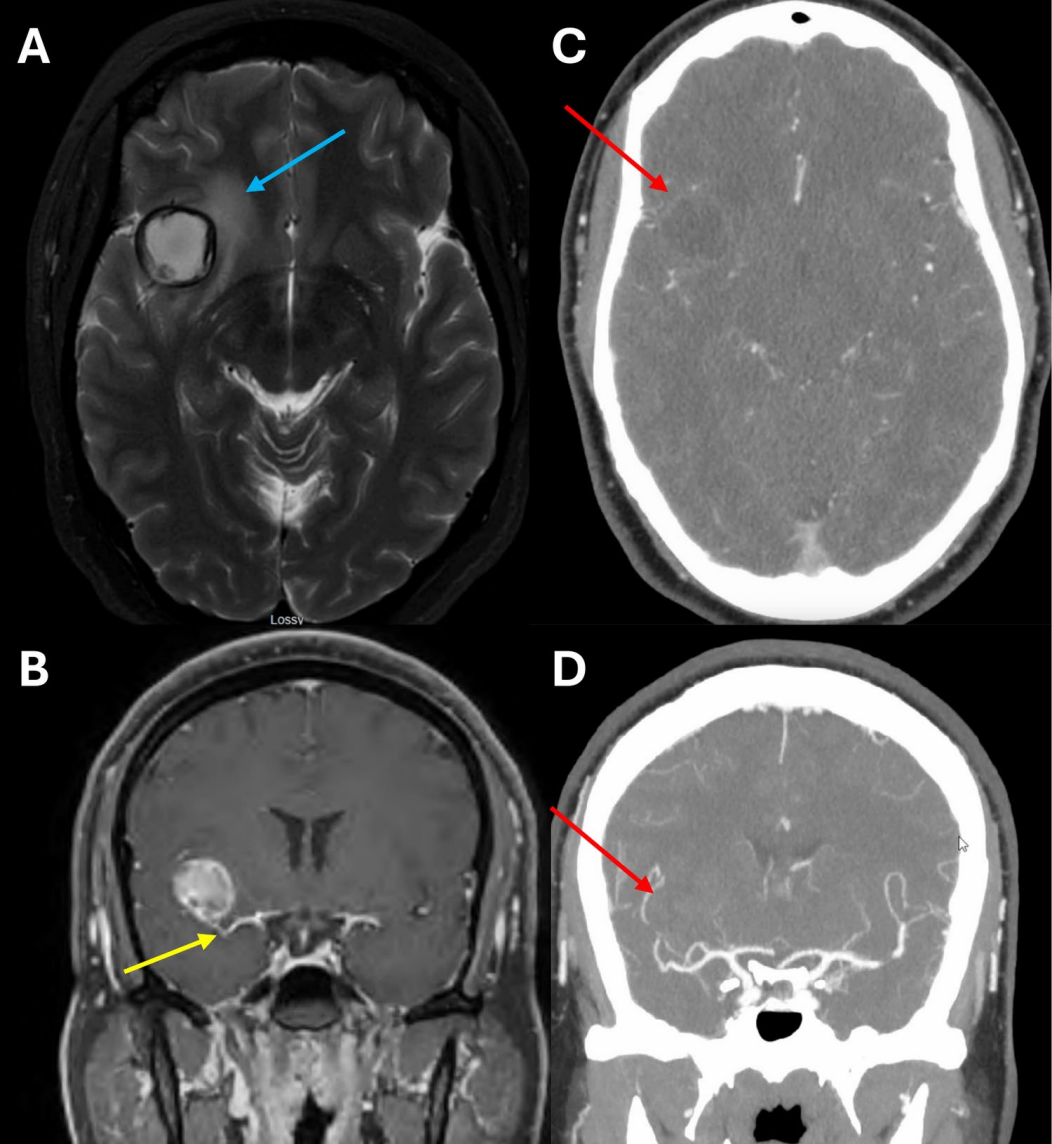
Pre-operative brain imaging demonstrates perilesional edema (blue arrow) on axial T2- weighted MRI of the brain (A), and post-contrast T1-weighted MRI (B) reveals involvement of the distal MCA bifurcation (yellow arrow). Axial (C) and coronal (D) CTA show no evidence of vascular irregularity (red arrows)
